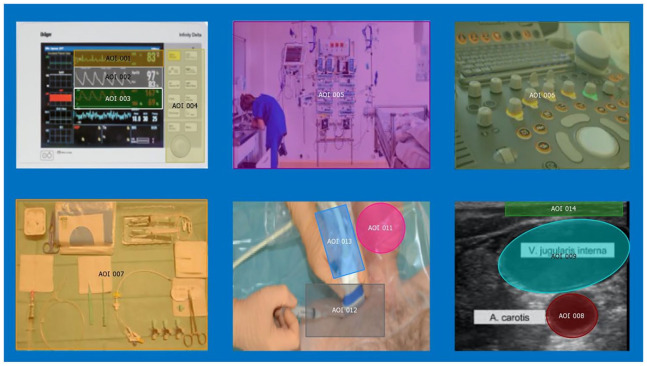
Reference map showing all areas of interest (AOI). AOI 1 = heart rate, AOI 2 = oxygen saturation, AOI 3 = invasive blood pressure, AOI 4 = monitor control panel, AOI 5 = surrounding equipment, AOI 6 = ultrasound control panel, AOI 7 = CVC preparation table, AOI 8 = carotid artery on ultrasound image, AOI 9 = jugular vein on ultrasound image, AOI 10 = white space (irrelevant, not shown), AOI 11 = patient’s neck, AOI 12 = cervical puncture location, AOI 13 = ultrasound probe, AOI 14 = skin insertion site on ultrasound image.
